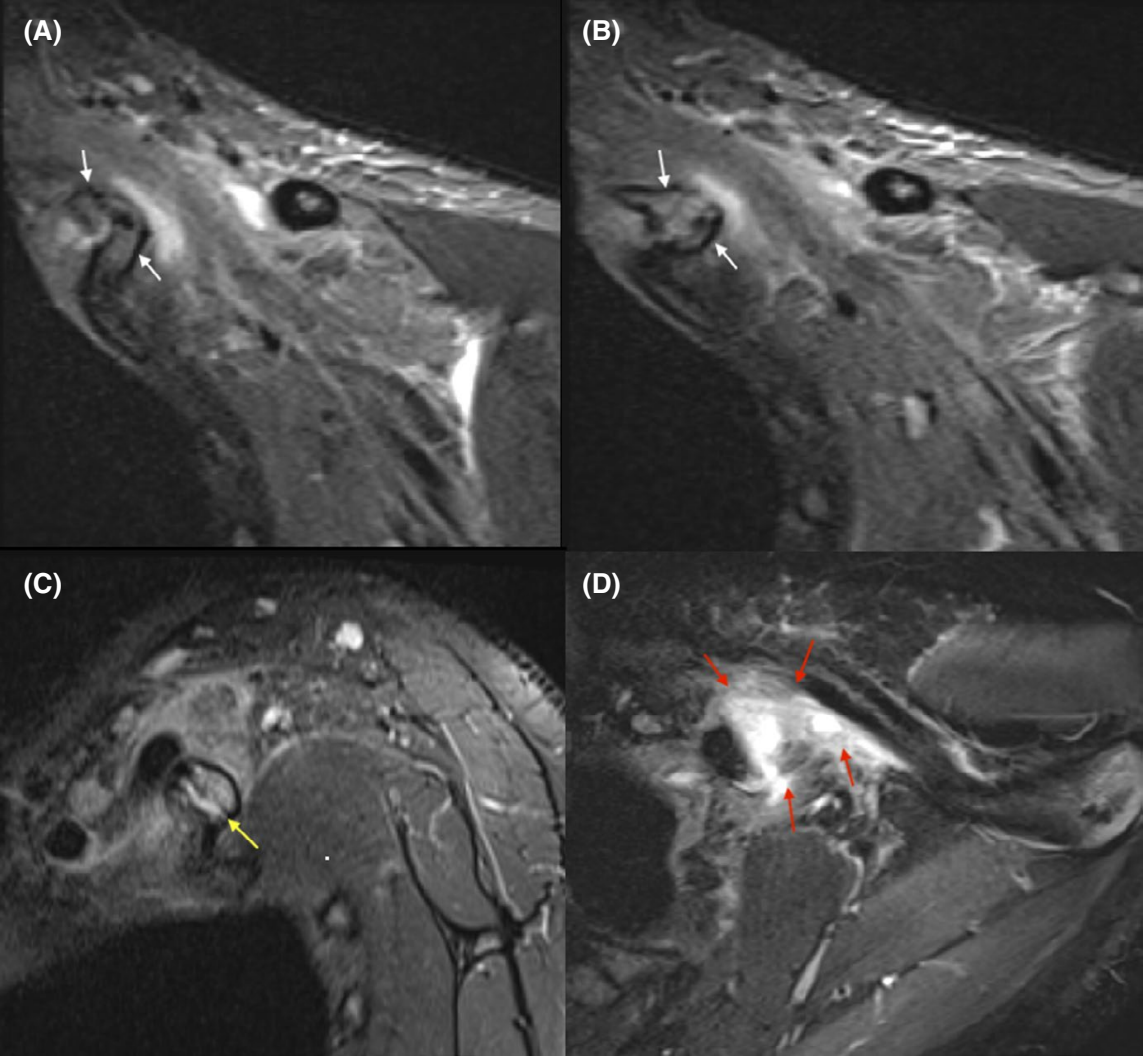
MRI of the left shoulder. (A and B) Coronal STIR images show marrow edema at both ends of the pseudoarthrosis involving the cervical and adjacent first ribs (white arrows). (C) Sagittal and (D) axial STIR images show a trace of fluid in the pseudoarthrosis region (yellow arrow) with surrounding soft tissue edema associated with small hematoma/fluid collection (red arrows)
